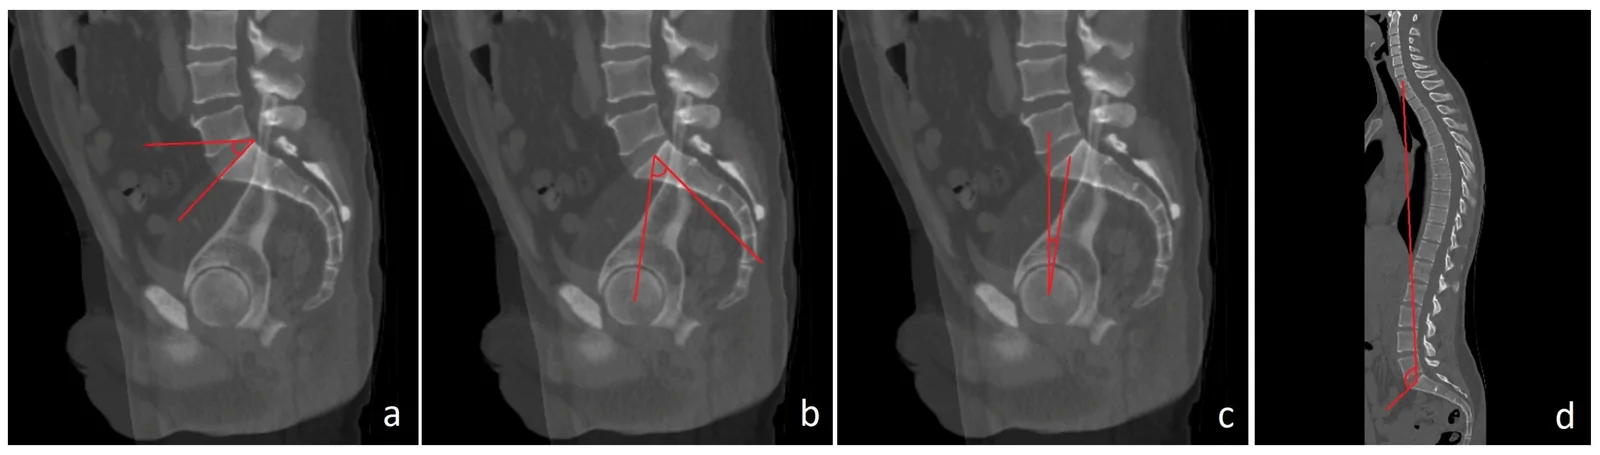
Sagittal lumbosacral computed tomography images obtained by merging two sagittal sections passing through the midline and the center of the femoral head show the measurement methods for sacral slope (a), pelvic incidence (b) and pelvic tilt (c), respectively. The method for measuring the spinosacral angle is shown in the sagittal computed tomography image of the vertebral column (d) passing through the midline. Red lines indicate the reference lines from which each angle was measured.
